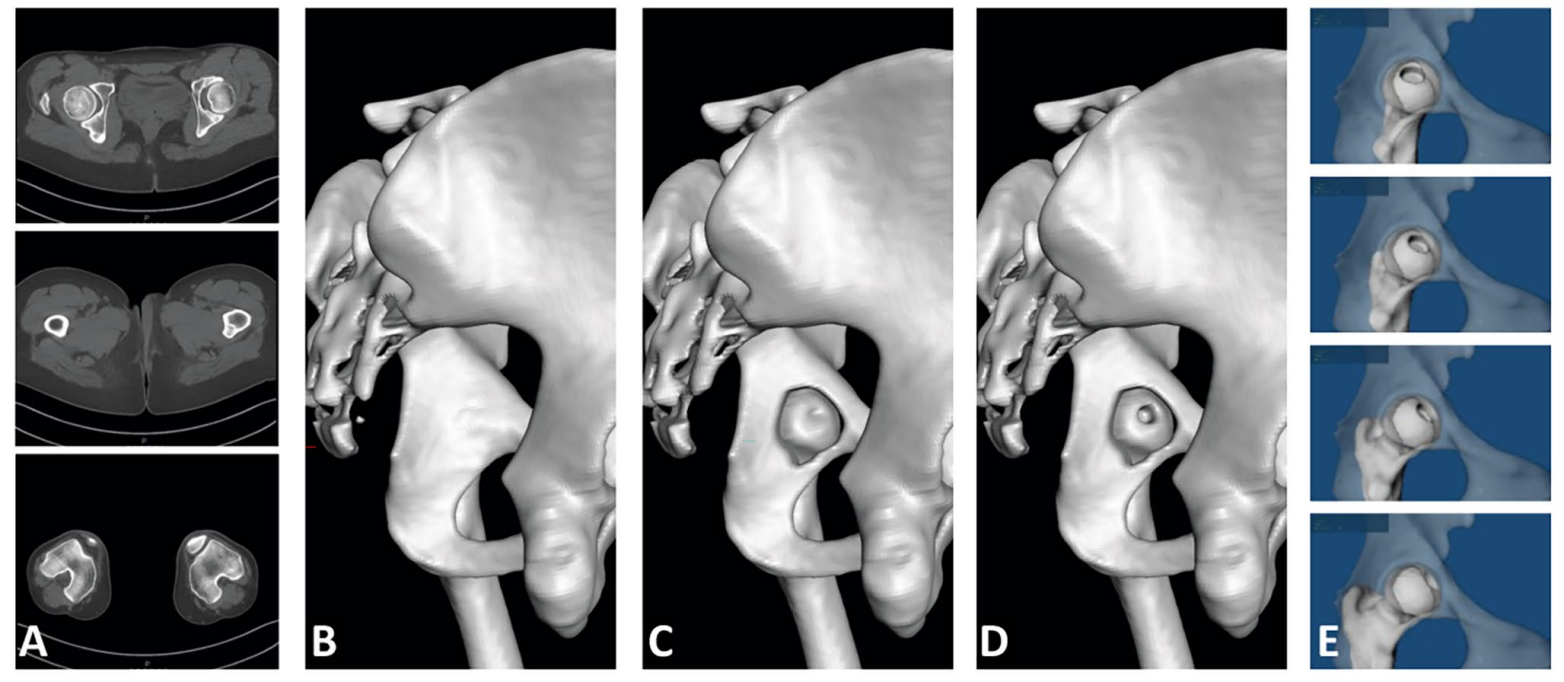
(A) Based on CT of the pelvis and distal femur; (B) we created a 3D model; (C) The acetabular fossa was digitally removed; (D) the fovea was identified and manually exaggerated; (E) finally, the model was subjected to standardized movements within physiological range of motion and the foveolar tracking pattern was determined
